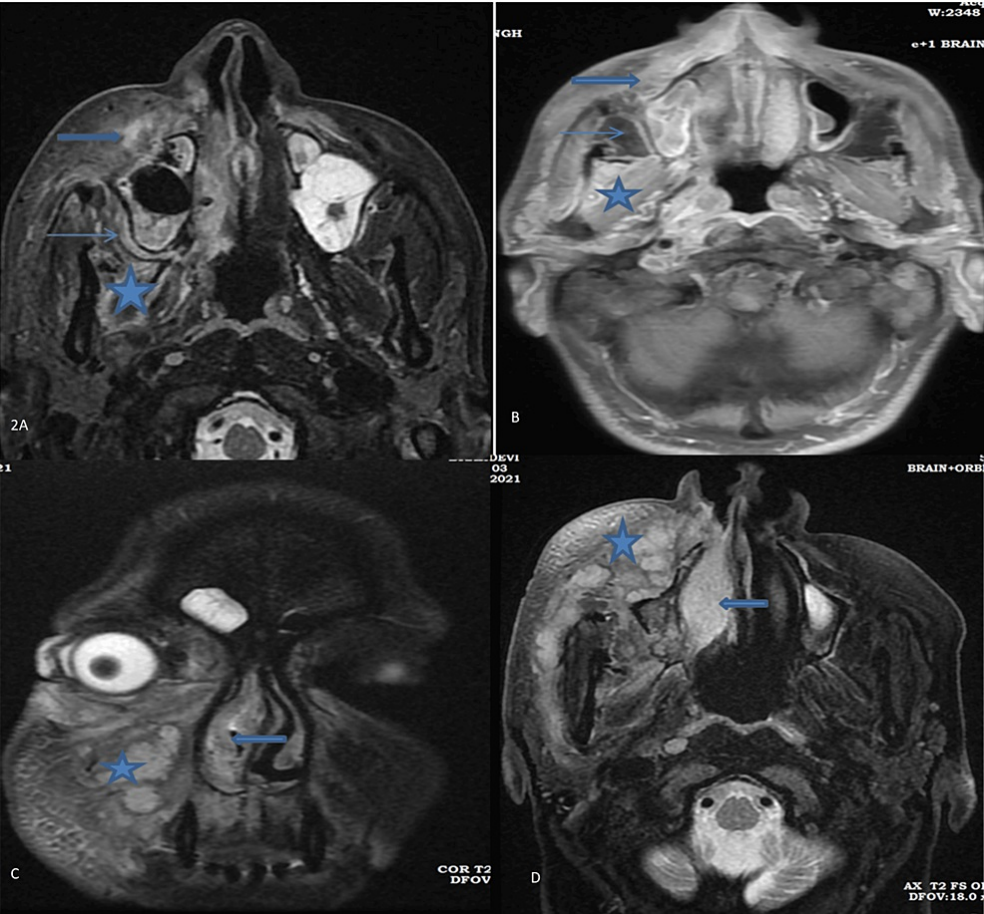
MRI of a 48-year-old male with extension fungal infection in soft tissue around paranasal sinuses. (A) Axial T2WFS reveals hyperintensity in the bilateral (right > left) preantral area (thick arrow) and right retroantral area (thin arrow), along with hyperintense infratemporal muscle (asterisk). (B) Axial T1FS contrast enhancement highlights areas involved in T2FS. (C) Coronal T2FS and (D) axial T2FS demonstrate the extension of infection in the preantral, premaxillary area (asterisk), and hyperintense inferior turbinates (thick arrow).
